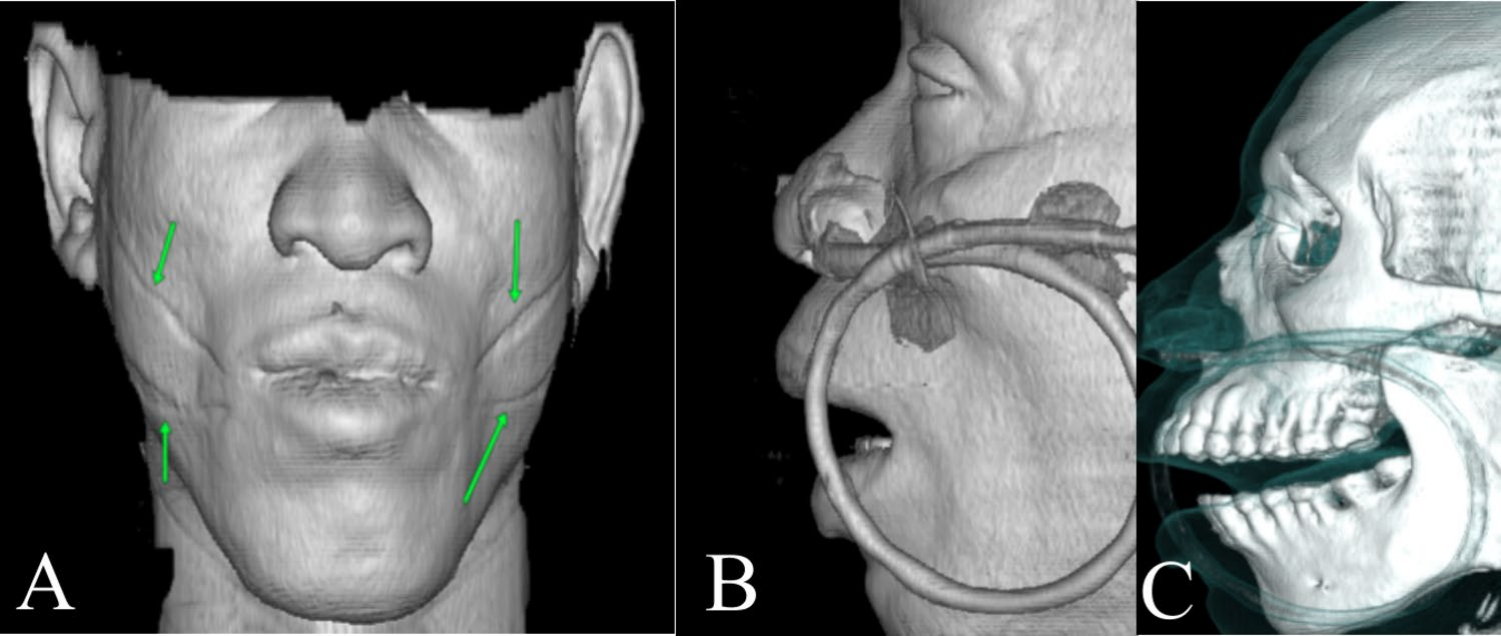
A subject with tribal mark denoted by green arrows. (A) Facial tribal marks denoted by green arrows (B) Naso-gastric tube (C) Naso-gastric tube in a CT bony window also showing soft tissues
